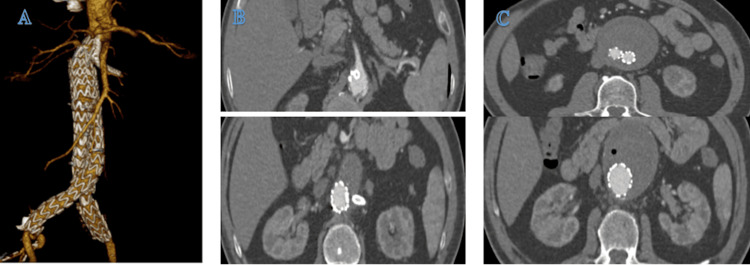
CT scan taken at the one-month follow-up appointment, demonstrating the post-procedure status of the abdominal aortic aneurysm A) Post-intervention status. B-C) Position of the chimney branch and stent-graft positioned without any observed endoleaks.
